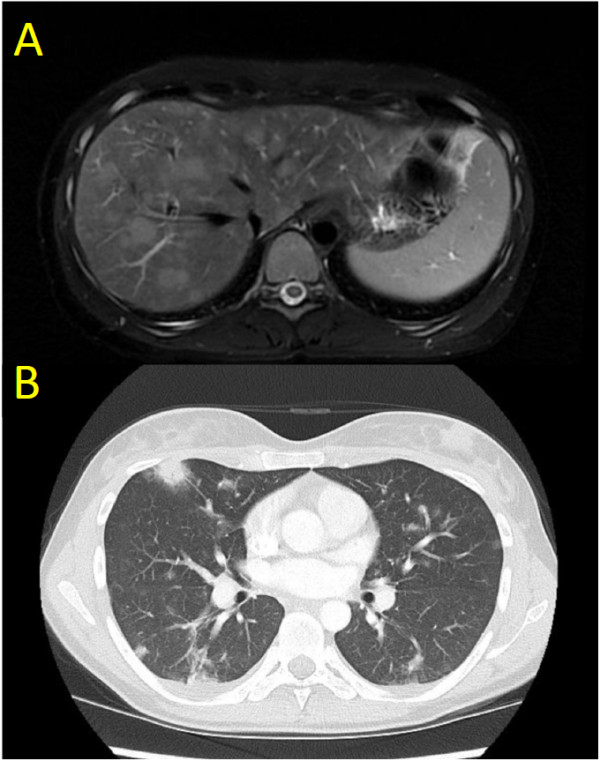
Diagnostic imaging features of lymphomatoid granulomatosis in our case. A) T2 weighted axial magnetic resonance (MR) image showing hyperintense lesions in the liver. B) CT axial image showing irregular bilateral pulmonary opacities with peripheral predominance and areas of ground-glass changes classic for lymphomatoid granulomatosis.
